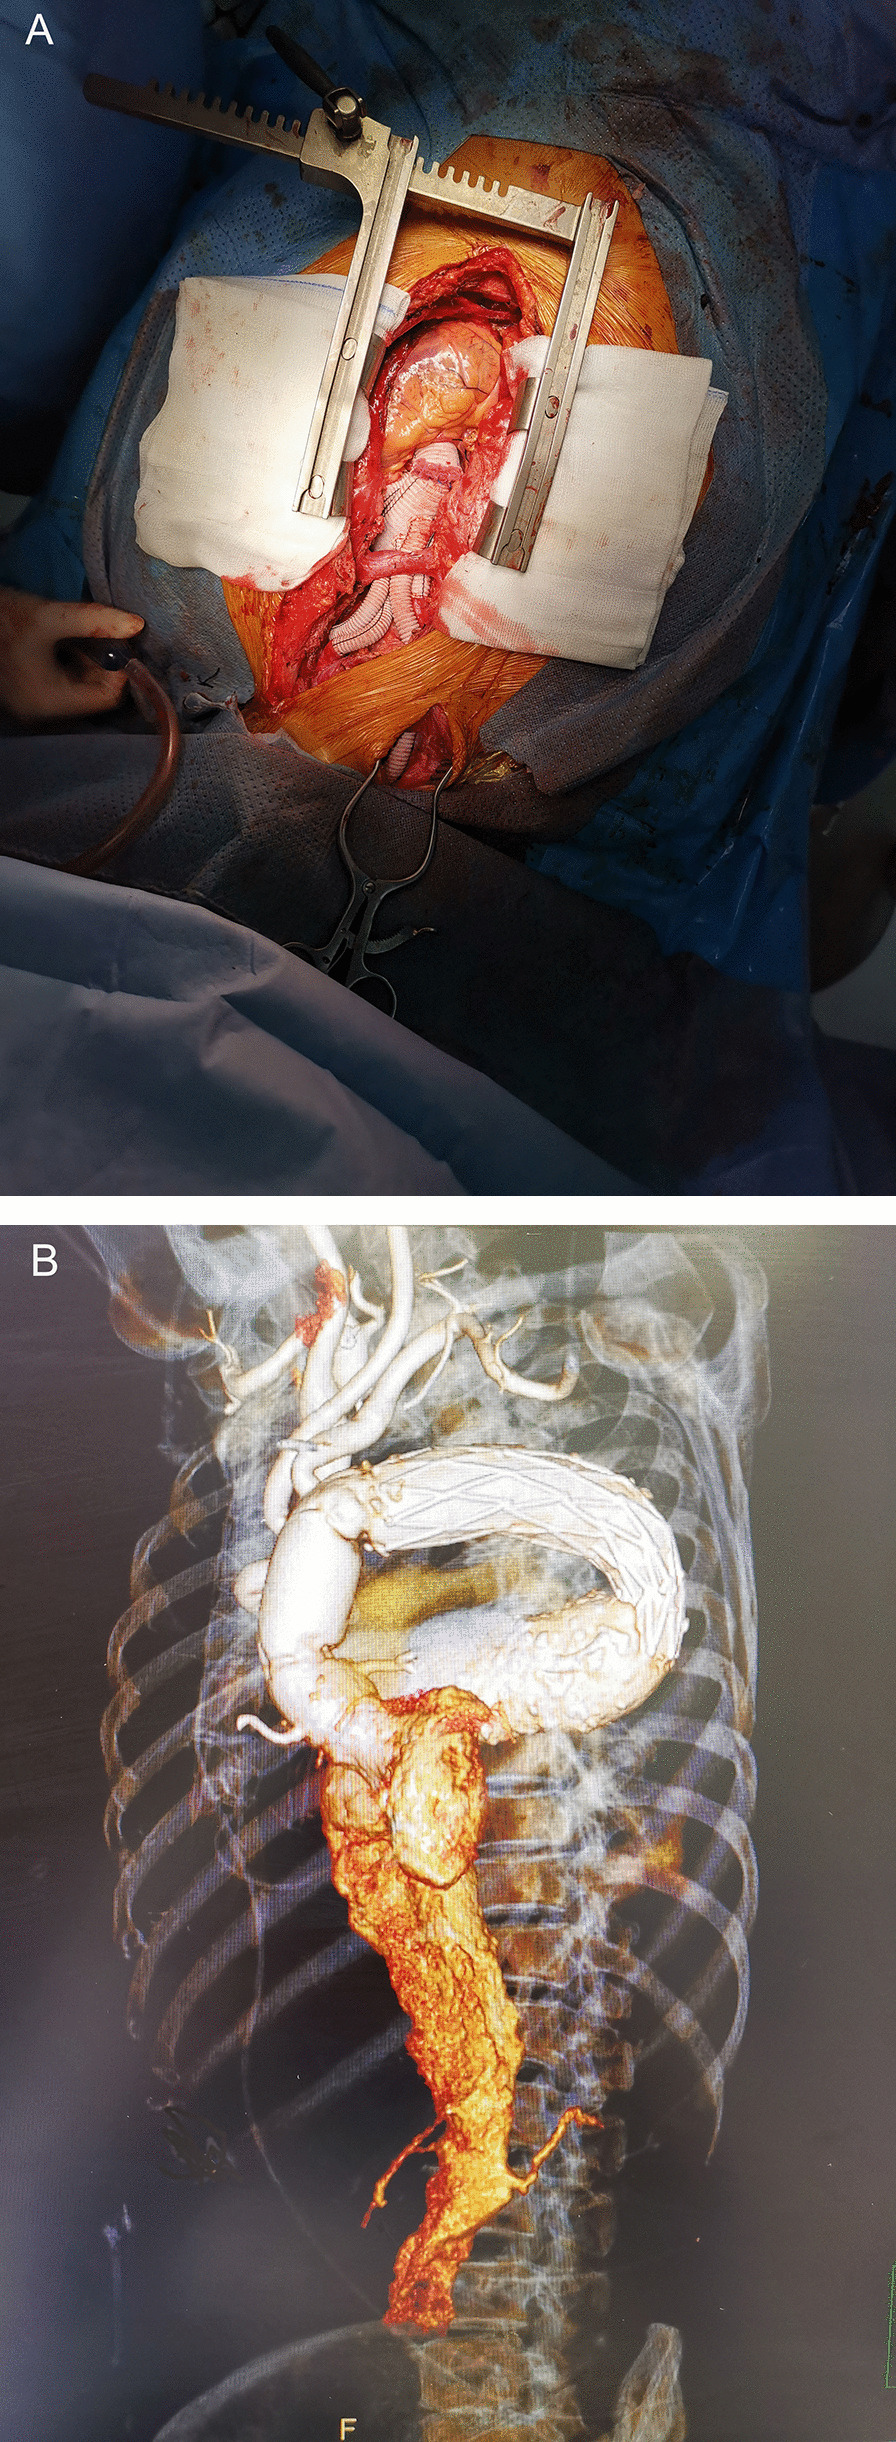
This patient successfully underwent the first-stage of Bentall and Sun’s procedure (A), and the postoperative CT (B) showed that the aortic root, ascending aorta, aortic arch, and proximal descending aorta were completely eliminated
